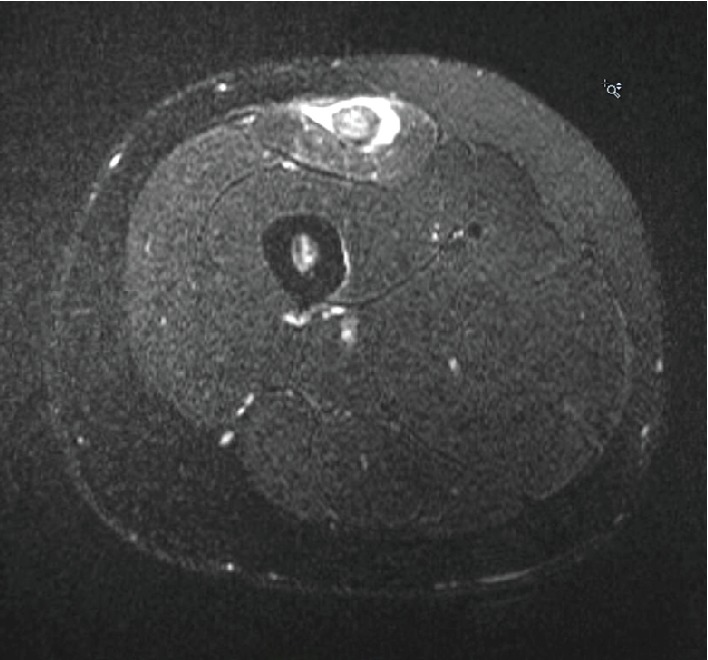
TSE T2 axial MRI image of the right femur without contrast demonstrating an acute degloving injury of the right rectus femoris.
